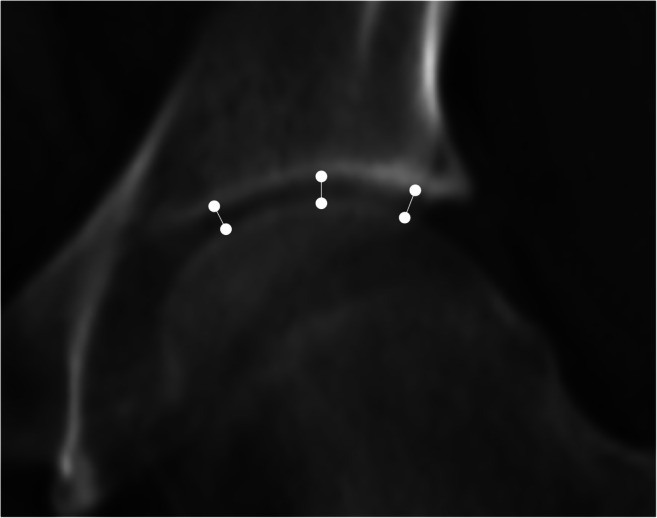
A 300-mm-thick coronal multiplanar reconstruction with an averaging of pixel intensities from a CT examination, as used to measure the joint space width. Location of the three sites of joint space width measurement is shown. From right to left: the superolateral, the apical, and the superomedial sites
